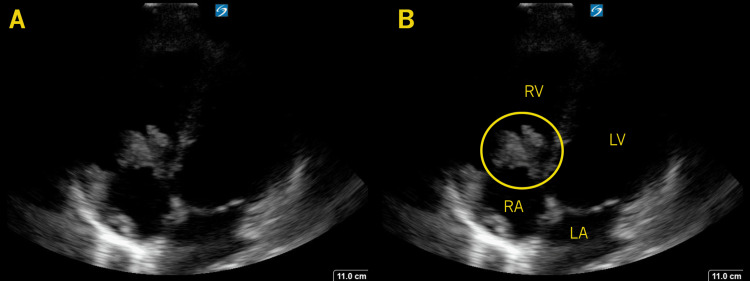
Tricuspid Valve Vegetation In this apical 4-chamber view, a large, echogenic mass (circle) is seen attached to the tricuspid valve. The right atrium (RA), right ventricle (RV), left atrium (LA), and left ventricle (LV) (B).
